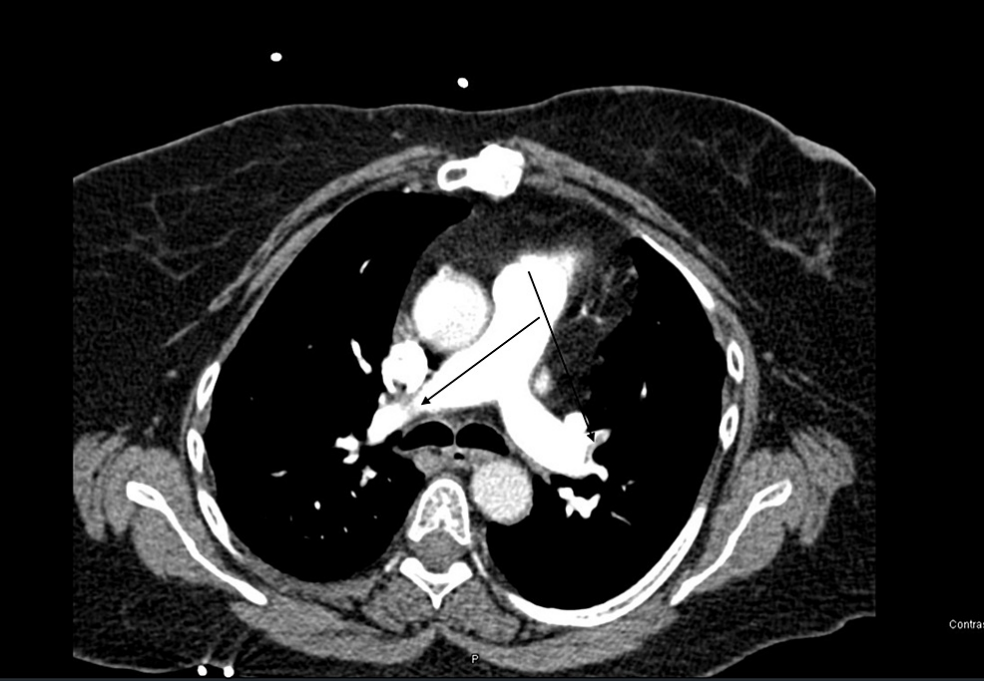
CT PE. Bilateral PE. PE, pulmonary embolism.
